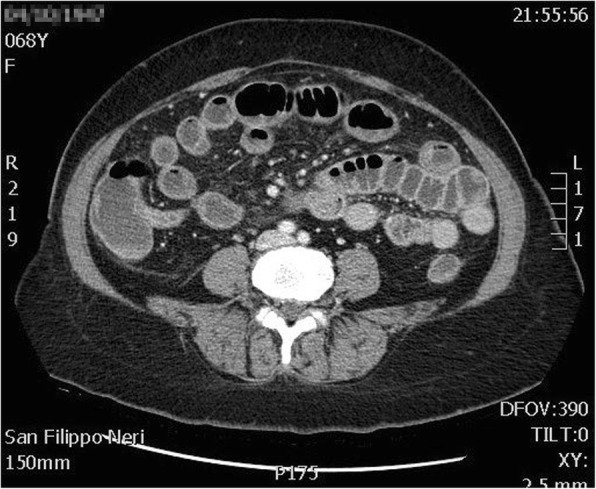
Computed tomography scan image. No abnormalities in the abdominal organs
